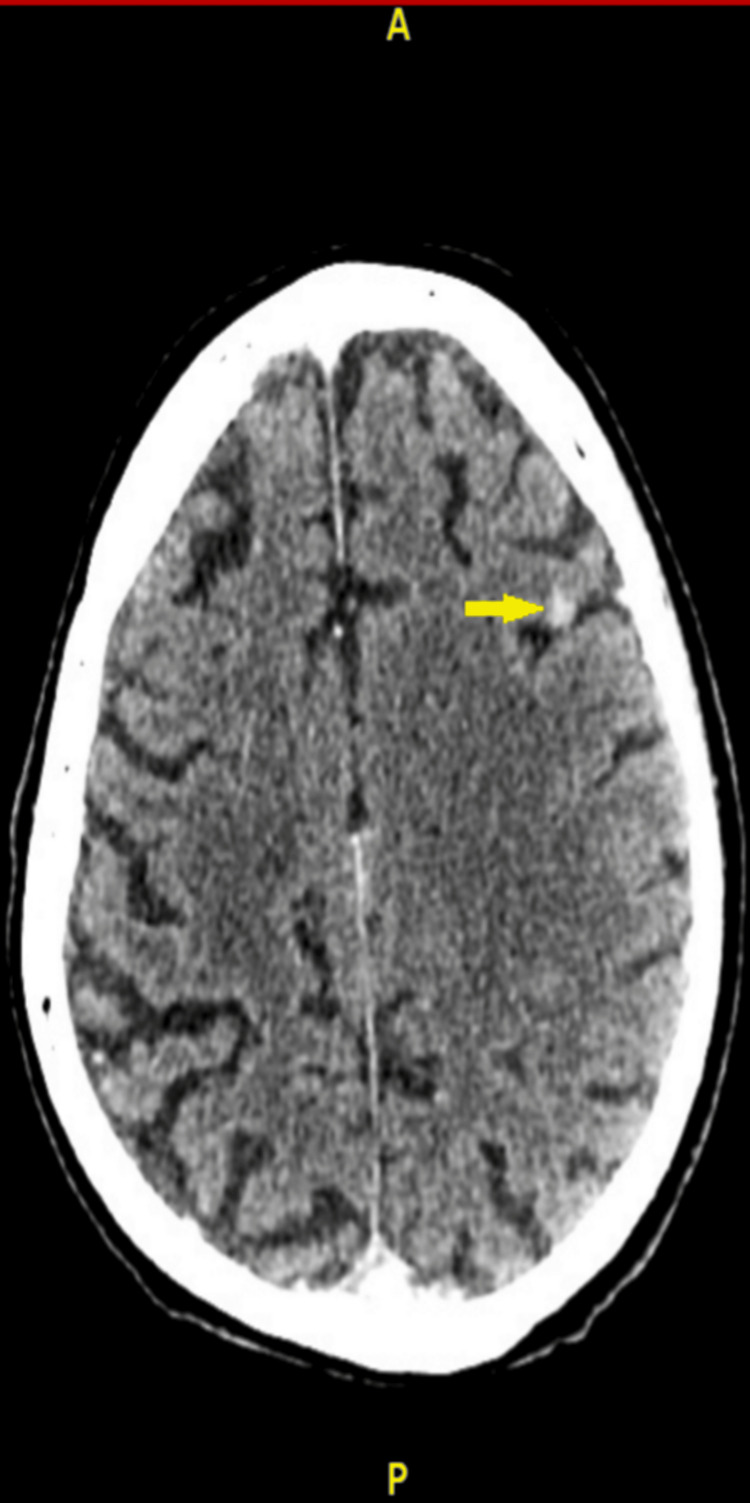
CT scan showing multifocal subcortical hyperdensity (yellow arrow).
